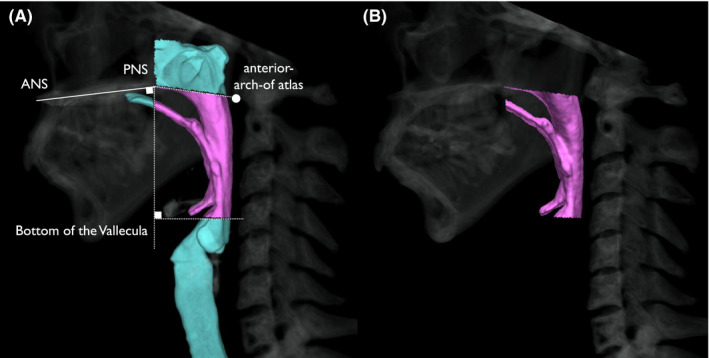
Definition of pharyngeal cavity. (A) Definition of pharyngeal cavity. Superior: the plane through the anterior nasal spine (ANS) and the posterior nasal spine (PNS), parallel to the infraorbital line. Diagonal: the plane inclined at an angle to the superior plane and passing through the inferior border of anterior arch of atlas (C1). Anterior: the plane perpendicular to the top plane and passes through PNS. Inferior: the plane through the bottom of the vallecula, parallel to the superior plane. (B) Depicted 3D‐CT image of pharyngeal cavity according to the definition
